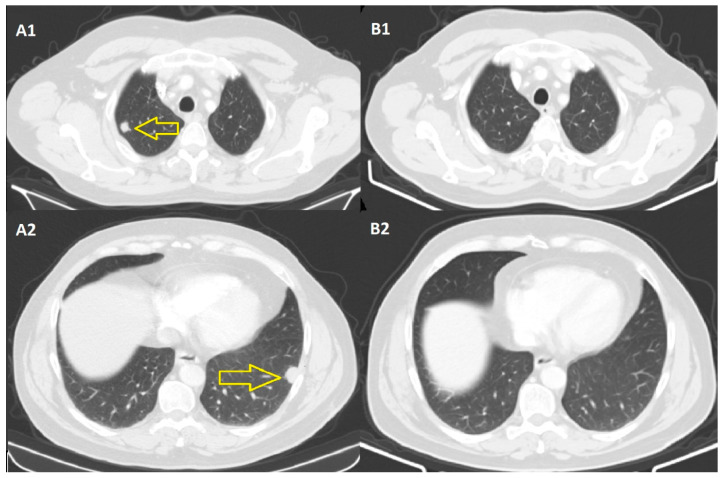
CT scans from December 2020 (A1,A2), where pulmonary metastases (yellow arrows) were evident on the CT images, compared to the CT scans from September 2021 (B1,B2), which indicate normal limits.
